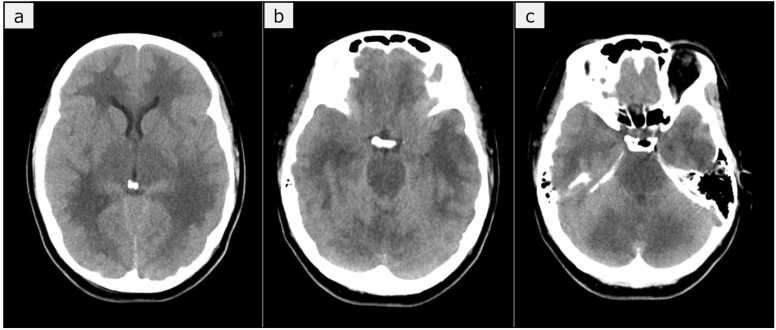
Head CT imaging 15 h after admission. The cerebrum and brainstem showed extensive low-density areas, leading to a diagnosis of diffuse brain swelling.
